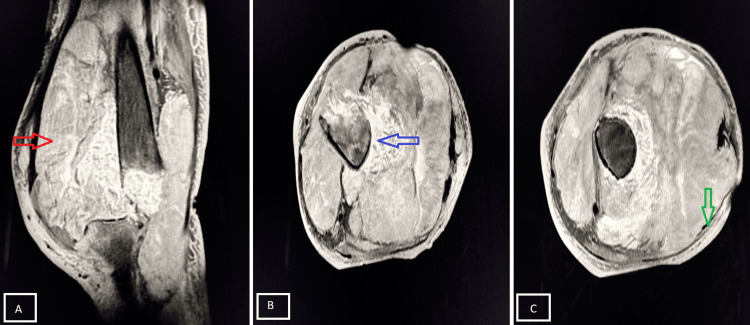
Malignant pleomorphic sarcoma around the knee joint Sagittal PD-FS MRI (A) demonstrates a heterogeneous soft tissue mass arising from the distal femur (red arrow) with cortical destruction and reactive bone formation, with extension into surrounding soft tissues. Axial PD-FS MRI (B) shows areas of high signal intensity (blue arrow), which represent myxoid components, while in the same imaging, a peripheral hypointense rim (green arrow) suggests the presence of a pseudo capsule. Findings are consistent with pleomorphic sarcoma. PD-FS, proton density fat-suppressed
